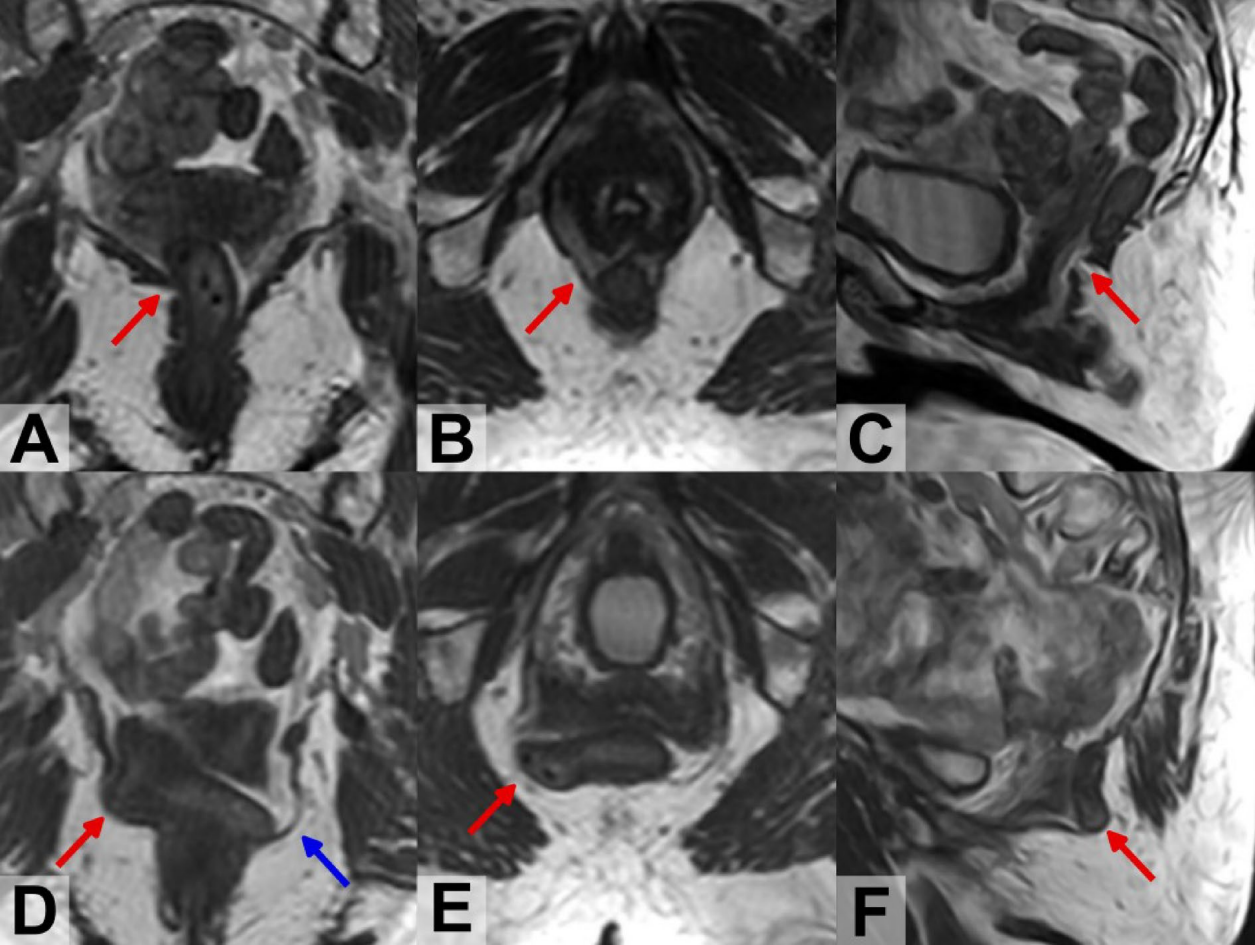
Example of differences in ICM in supine (A, B,C) and upright (D, E,F) position MR images from a 68-year-old with cystocele (POP-Q stage 3) and rectocele (POP-Q stage 2). Coronal image (A) in supine position at the level of the rectum showed a gap in the right iliococcygeus (red arrow) that can be seen in axial (B) and in sagittal (C), scoring as ICM minor damage. Coronal image (D) in up-right position at the level of the rectum showed a focal bulging in left (blue arrow) and right side of the ICM (red arrow). In axial (E) and sagittal (F) can be seen that the right damage is a herniation with rectum protrusion, scoring as major damage (red arrow).
